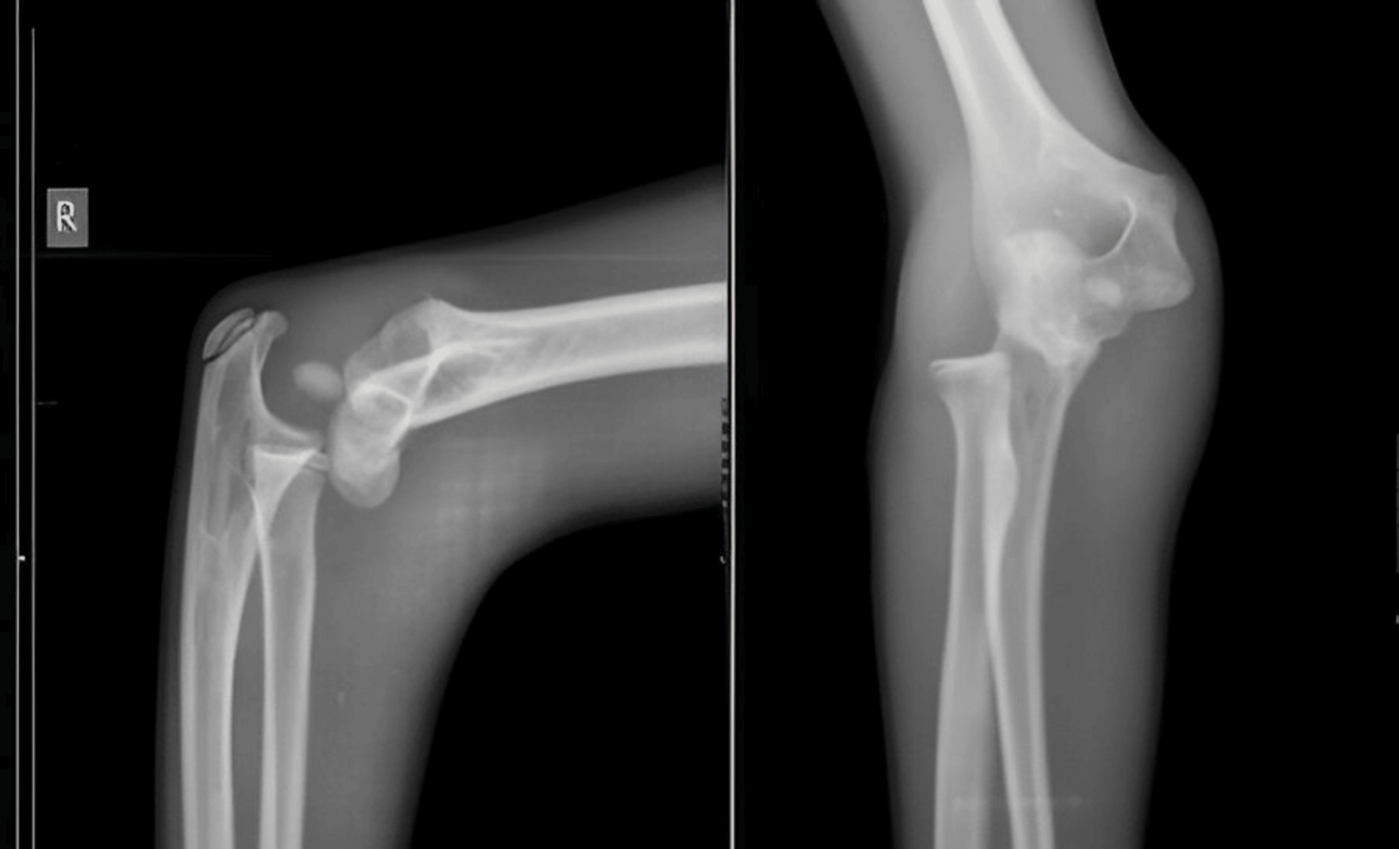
Preoperative X-ray of case 4 showing fracture dislocation of the right elbow
